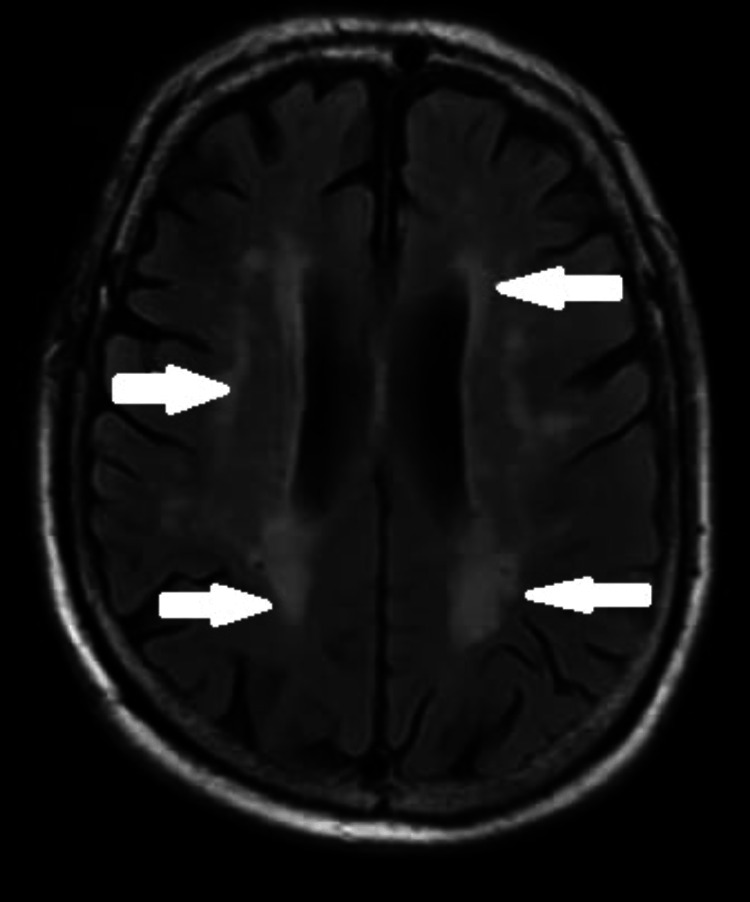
Hyperintense signal changes in the deep white matter in MRI T2 FLAIR image MRI - Magnetic resonance imaging; FLAIR - Fluid-attenuated inversion recovery
